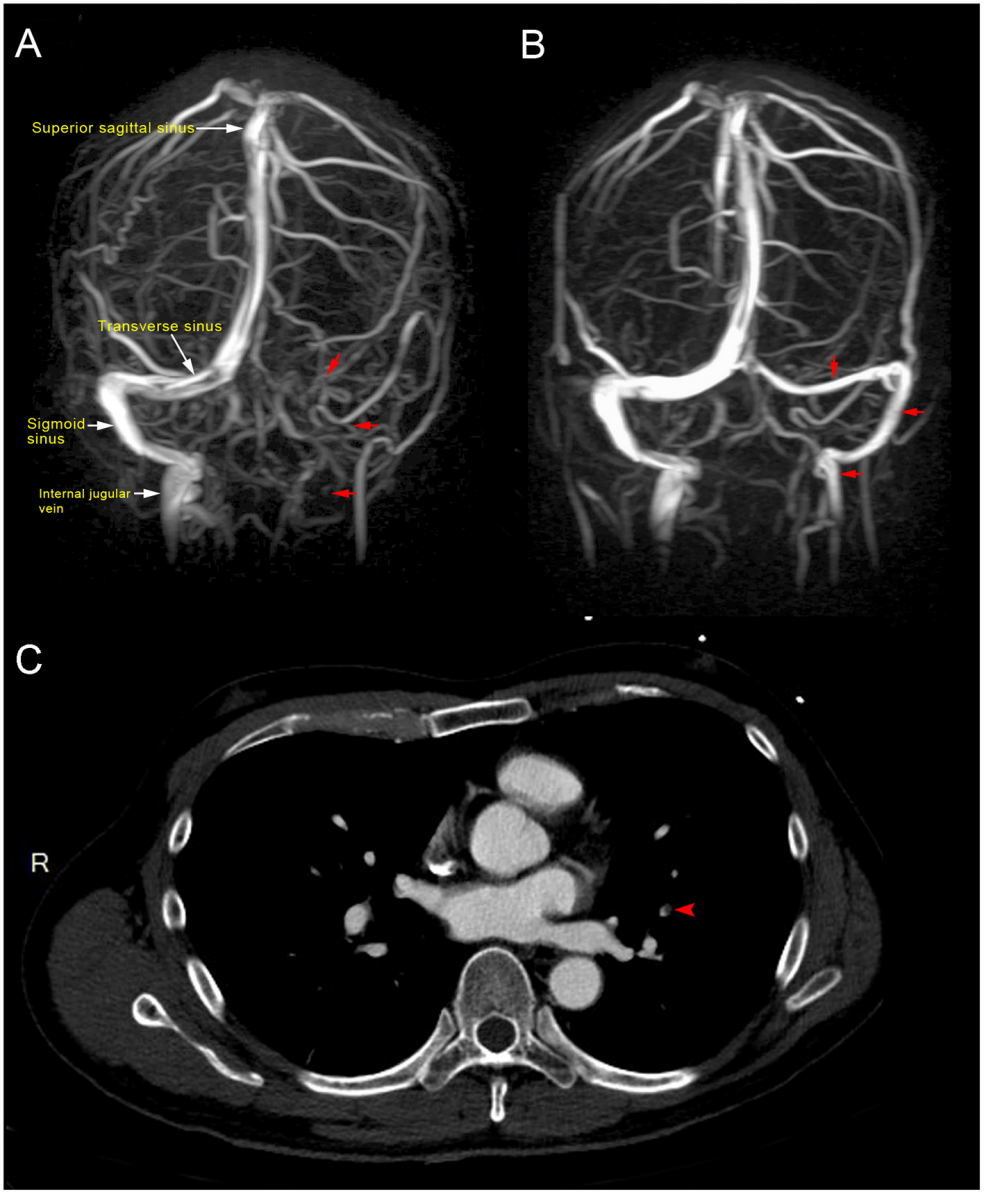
(A) Magnetic resonance venography showed loss of signal in the left transverse sinus, sigmoid sinus, and left distal internal jugular vein (red arrow), which were consistent with dural venous sinus thrombosis at 10 days post-vaccination. (B). Magnetic resonance venography after 2 months of direct oral anticoagulant (10 mg apixaban twice daily for initial 7 days, 5 mg twice daily for the following 2 months) showed patent left transverse sinus, sigmoid sinus, and left distal internal jugular vein (red arrow), indicative of recanalization and disease regression. (C) Computed tomography showed a filling defect in the left upper lung (red arrow), consistent with pulmonary embolism.
